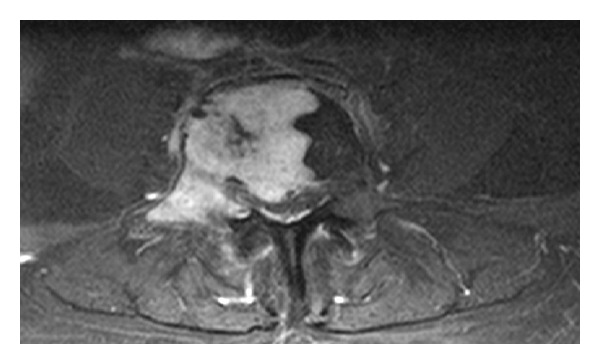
MRI lumbar spine: enhanced T1 weighted axial image. Enhancing, well-defined benign phosphaturic mesenchymal tumor in lumbar vertebra.
